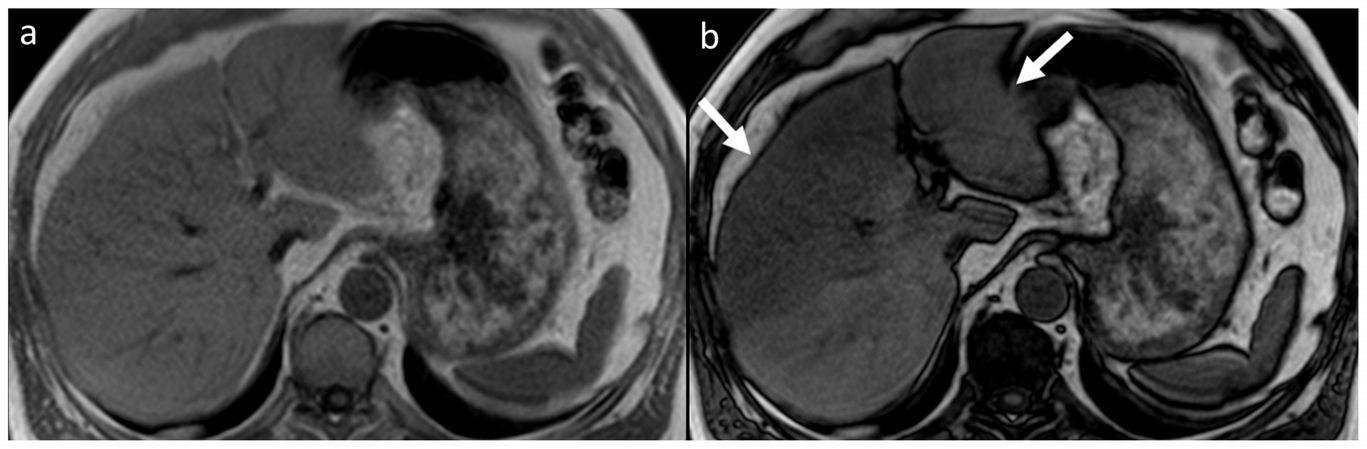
In-phase (A) and opposed phase (B) chemical shift MR images of a 51-year-old male demonstrate hepatic steatosis, consistent with areas of decreased signal intensity on an opposed-phase image. Steatosis is more pronounced it the left lobe and the anterior segments of the right liver lobe (arrows in B).
